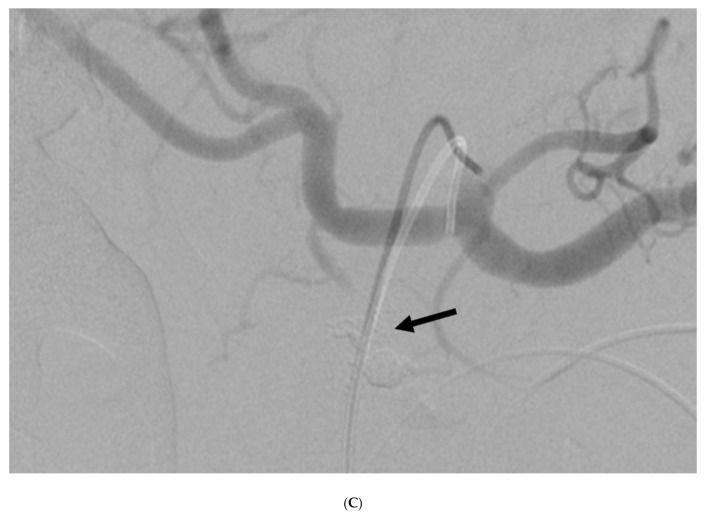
Transcatheter arterial embolization with N-butyl cyanoacrylate. Angiographic images of a 73-year-old man who underwent distal pancreatectomy for pancreatic body cancer. Bloody fluid was detected in the drainage tube 13 days after surgery, and contrast-enhanced computed tomography was performed. (A) A pseudoaneurysm (indicated by the arrows) was identified in the gastroduodenal artery by contrast-enhanced and three-dimensional CT (left and right images, respectively). (B) The celiac axis angiogram revealed a pseudoaneurysm in the gastroduodenal artery. A microcatheter could not be inserted into the distal site of bleeding, and so 0.6 mL of a 1:3 mixture of N-butyl cyanoacrylate/iodized oil was injected from the proximal site of hemorrhage. (C) Hemostasis was achieved after embolization with N-butyl cyanoacrylate (indicated by the arrow). There were no signs of recurrent bleeding or complications after transcatheter arterial embolization.
